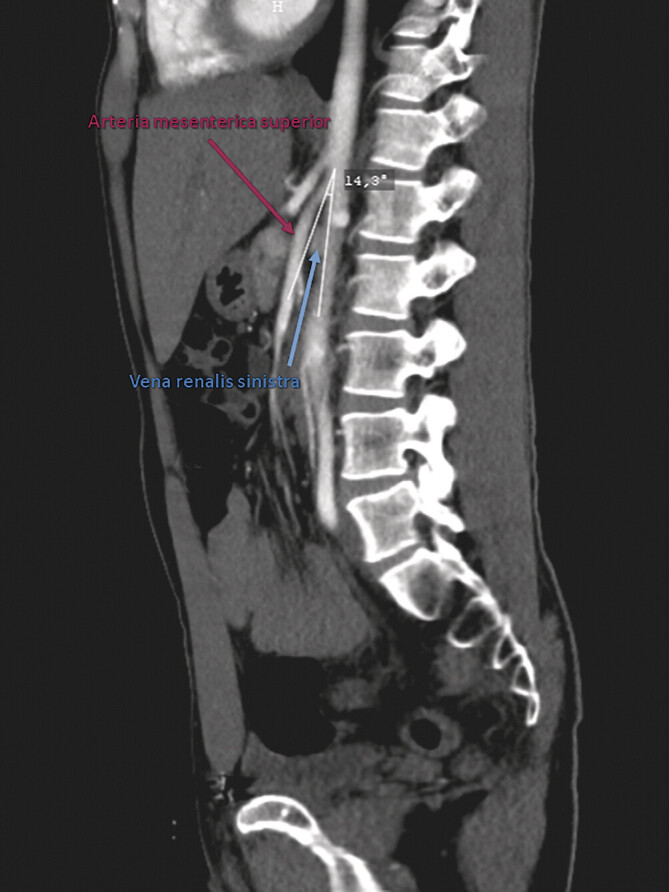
Angio-CT-Scan (sagittale Rekonstruktion mit arterieller Kontrastmittelphase): Kompression der linken im Querschnitt abgebildeten Nierenvene zwischen der A. mesenterica superior (ventral) und der Aorta abdominalis (dorsal) bei signifikant reduziertem Aortomesenterialwinkel (14,3°).
